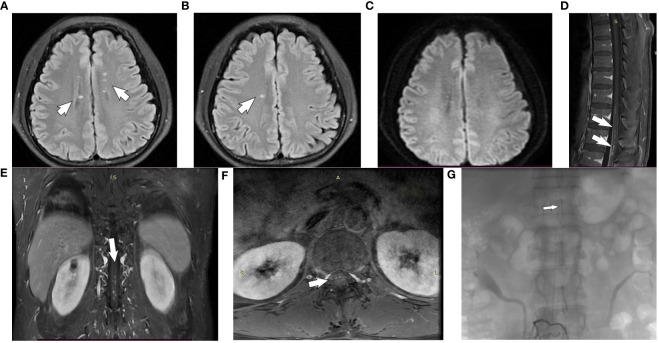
Brain and spinal MRI of a subject with autoimmune GFAP astrocytopathy. (A, B) The cranial MRI T2 FLAIR sequences: multiple patchy abnormal signals beside the anterior and posterior horns of the bilateral lateral ventricles and in the center of the semiovals(white arrow). (C) Diffusion Weighted Imaging(no high signal seen). (D–F) The gadolinium-enhanced T1-weighted image shows patchy enhancement of thoracic spine 11- lumbar spine 1 (white arrows). (G) Spinal digital subtraction angiography: the third lumbar artery on the right side of the vertebral canal to the supply of the anterior spinal artery in the spinal canal about the thoracic 10 position(white arrows).
